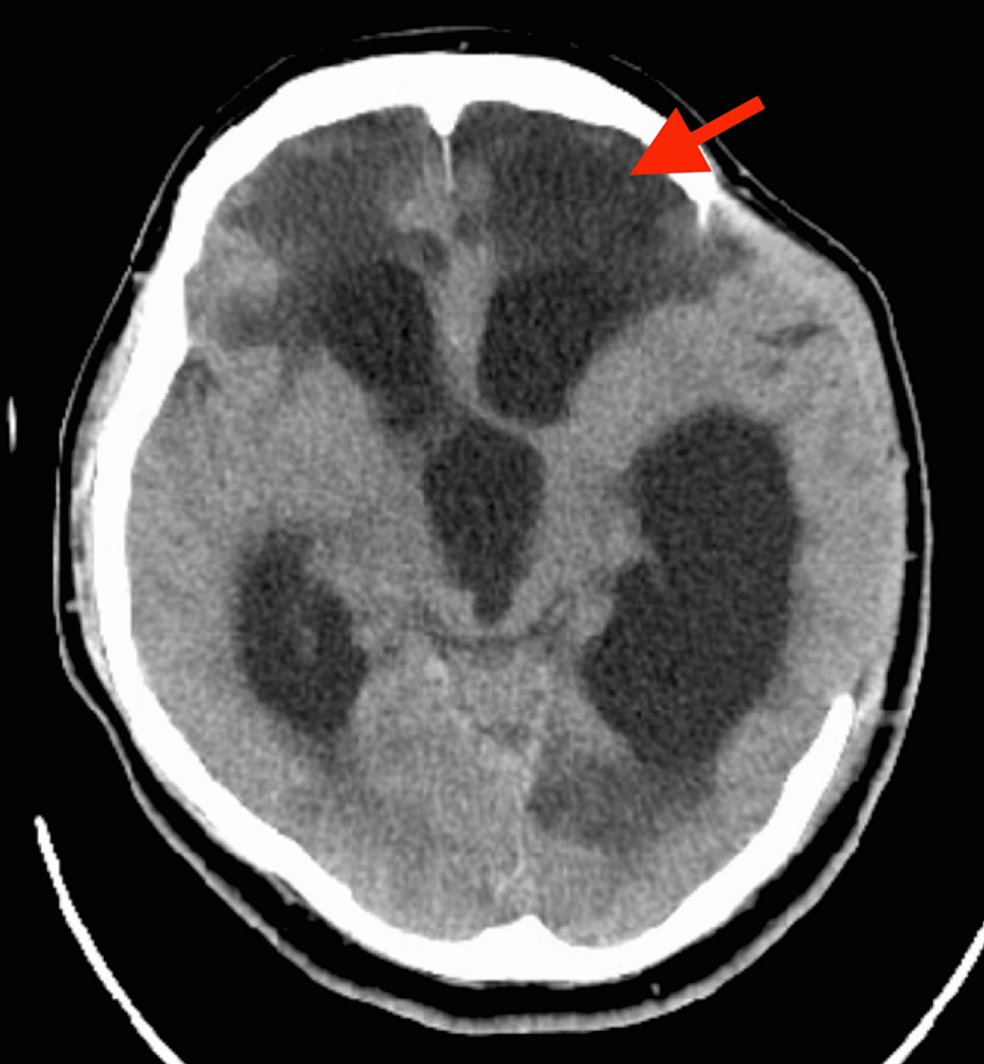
Axial CT head three months after injury, demonstrating ventriculomegaly with transependymal flow (red arrow) and mild herniation of brain parenchyma through craniectomy defect
